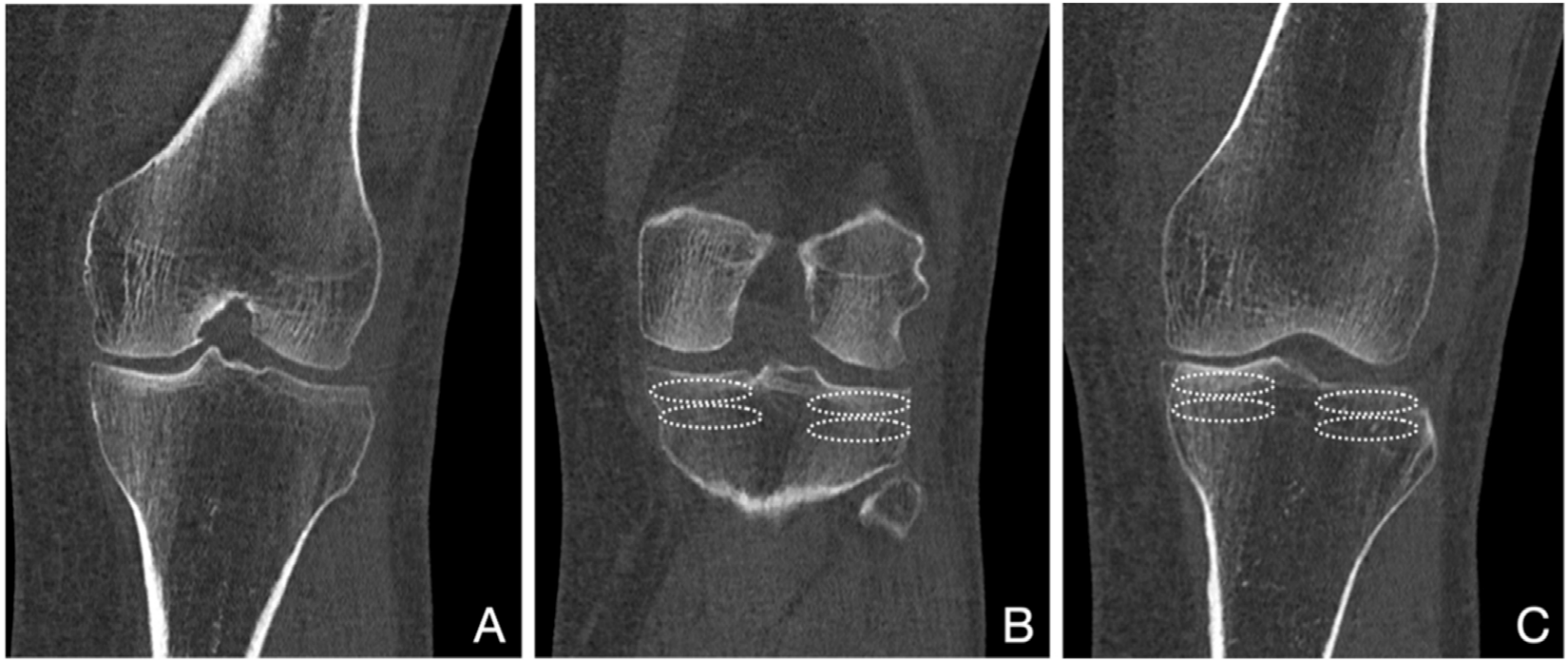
Coronal views of a CT scan of the tibial plateau and distal femur. (A) shows the midline cut were the lines a, b and c of Figure 1 meet. (B) shows three slices back, with the ellipses to measure HU in the posterolateral and posteromedial subchondral and metaphyseal regions. (C) shows three slices forward, with the ellipses to measure HU in the anterolateral and anteromedial subchondral and metaphyseal regions.
